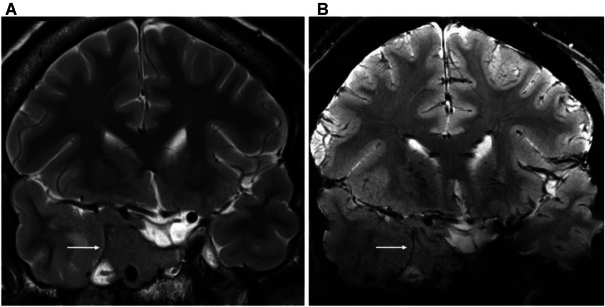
These images demonstrate how 7-T MRI provides more anatomical details of human brain than 3-T MRI. The white arrow denotes a homogenous sella macroadenoma. Comparison of 3 T (A) and 7 T (B) coronal T2-weighted images demonstrates far more detail of microvessels within the mass indicative of neovascularity, which is a known pathological feature of some macroadenomas (these images are adapted from Obusez *et al*., 2018).
